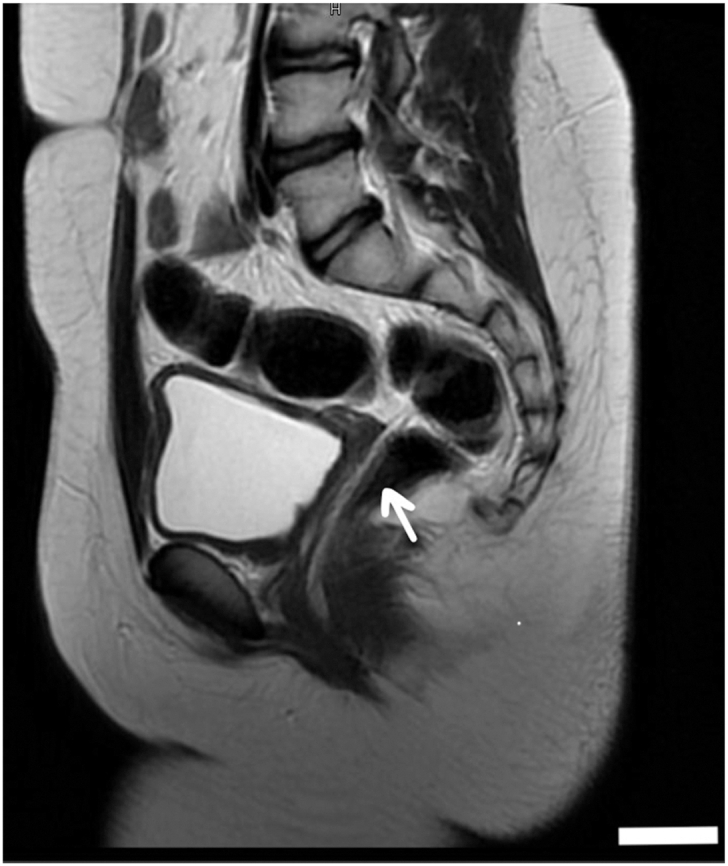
Sagittal T2-weighted MRI demonstrating an absent or hypoplastic uterus and *Upper* vagina (arrow), located posterior to the bladder.The bladder appears normal in size and shape. The bowel loops and spine alignment are unremarkable.
